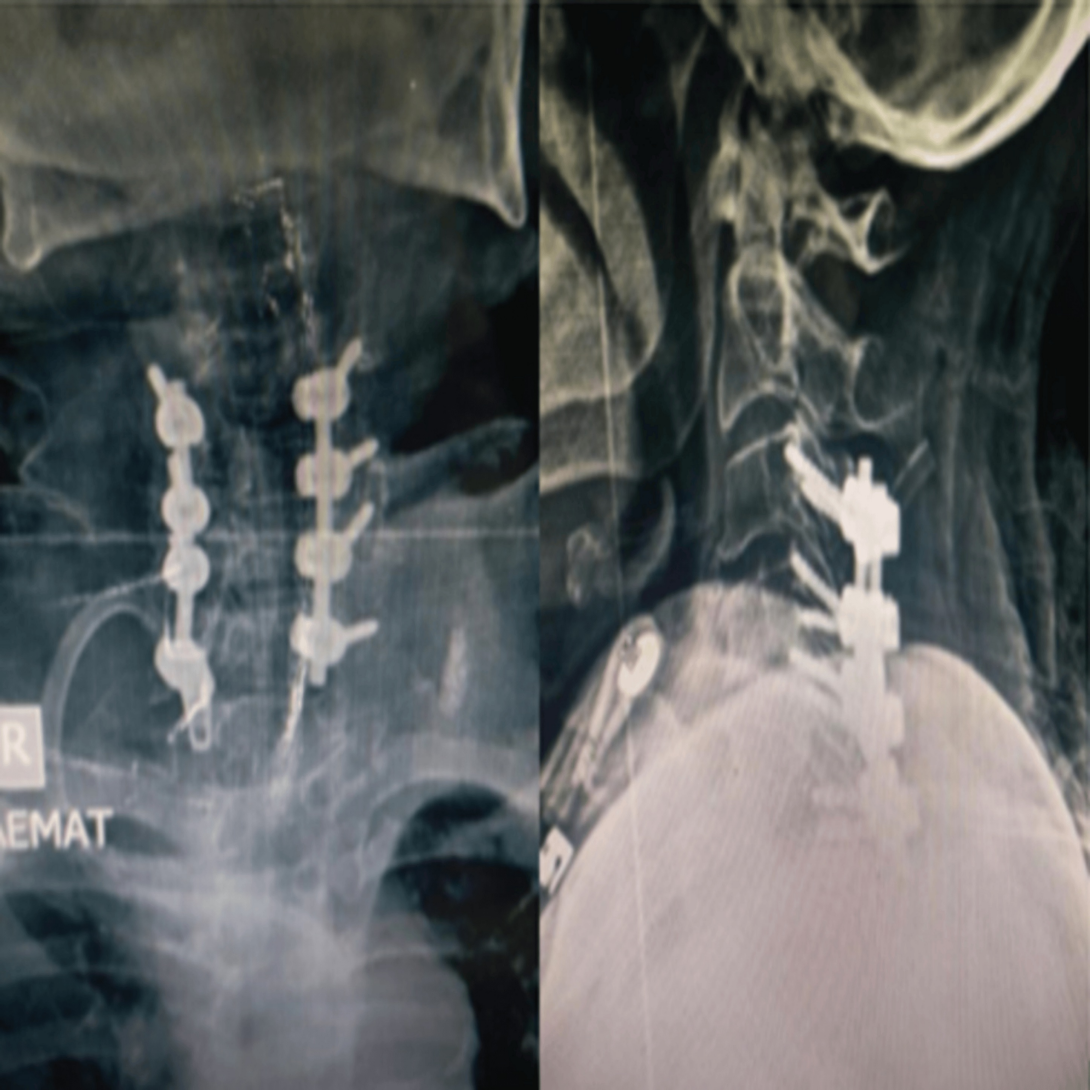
Postoperative plain X-ray of the cervical spine (A) Anteroposterior (AP) view and (B) lateral view showing C3-C6 decompression and lateral mass fixation.
